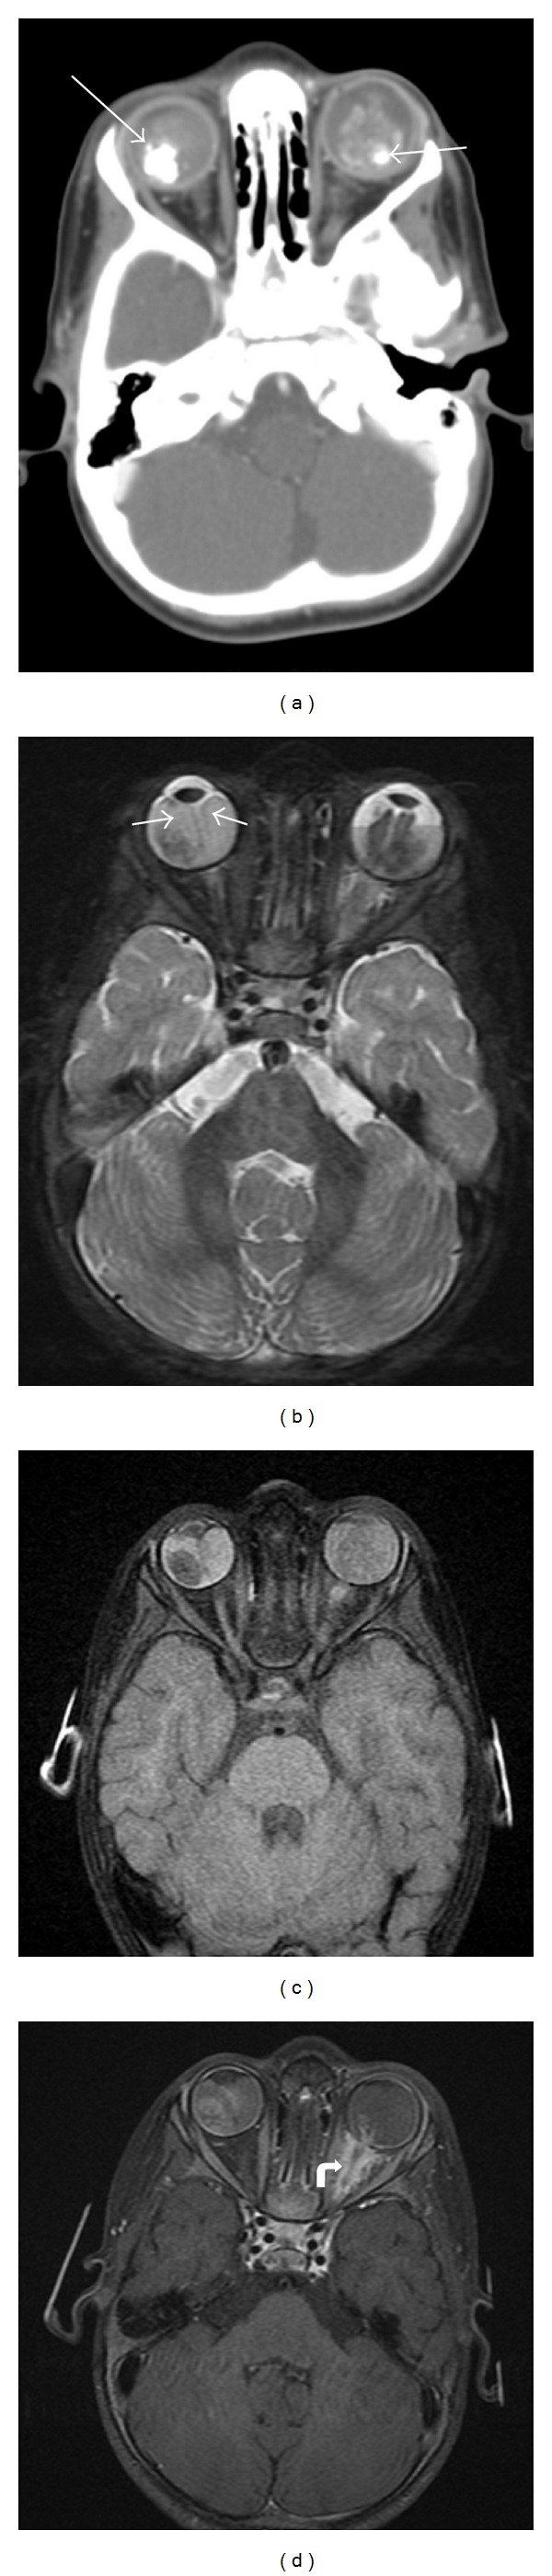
17 months old diagnosed with bilateral retinoblastoma requiring systemic chemotherapy. Axial contrast-enhanced CT through the orbits (a) demonstrates bilateral intraocular masses with coarse calcifications (arrows) and mild-to-moderate enhancement, diagnostic of retinoblastoma. MR axial T2-weighted image with fat-saturation (b) demonstrates curvilinear hypointensities in both globes, best visualized on the right, consistent with retinal detachment (arrows). Axial T1-weighted fat-saturated (c) and postcontrast T1-weighted fat-saturated (d) images show the bilateral intraocular masses with abnormal signal and enhancement in the intraconal left orbit along the optic nerve (curved arrow), which may represent a combination of postlaminar spread of tumor along the optic nerve and secondary perineuritis. This is not apparent on the CT scan.
